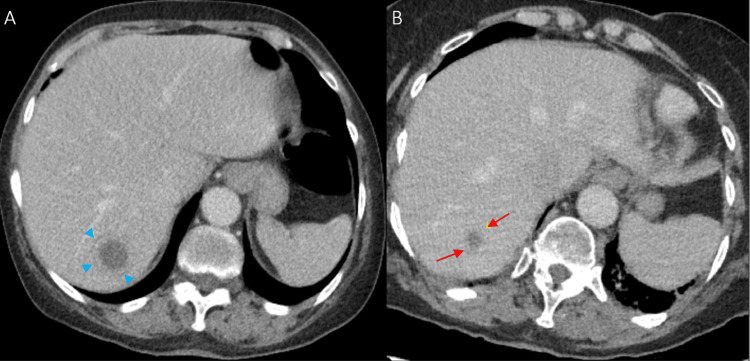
Axial contrast-enhanced computed tomography (CT) of the upper abdomen. A hypoenhancing round segment 7 hepatic lesion shows doubling in size (A) compared with the CT study obtained 3 weeks before (B, red arrows). It is surrounded by a thin hypoattenuating rim (blue arrowheads, A), after a narrow zone of enhancing parenchyma, demonstrating "double target sign." Findings are suggestive of a hepatic abscess.
